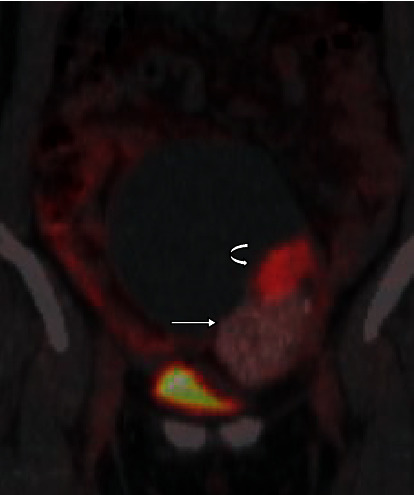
Coronal fused PET/CT image of the borderline Brenner tumor. The PET/CT shows a mild FDG uptake (SUVmax: 2.3) and calcification in the solid component (arrow), and moderate FDG uptake (SUVmax: 5.8) in the papillary tumor (curved arrow).
